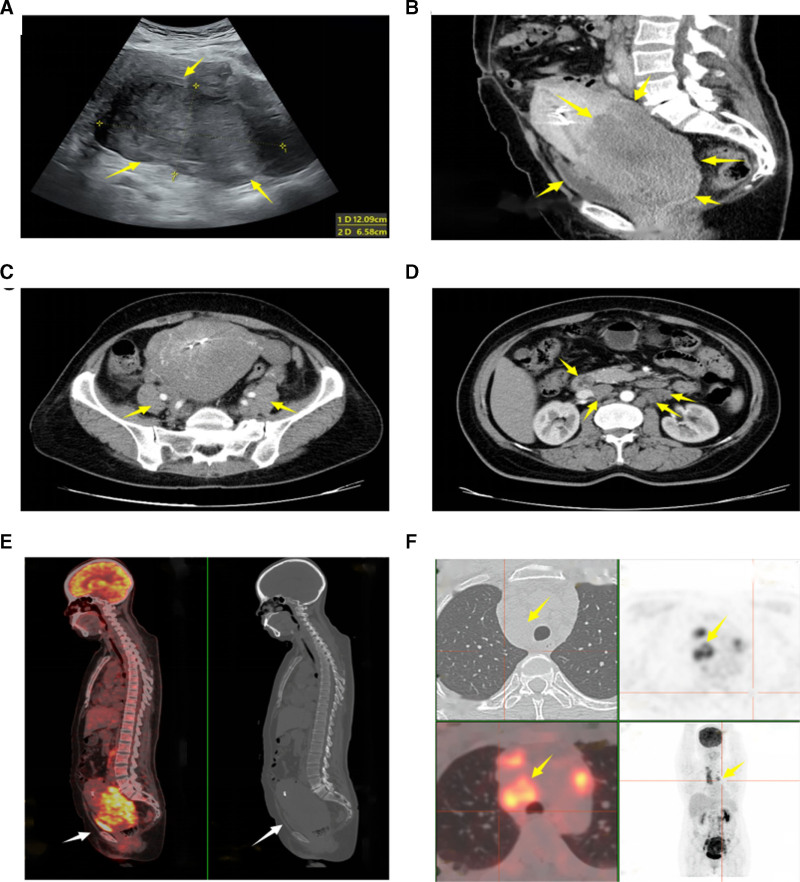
Imaging indicated the mass and lymph nodes. (A) Ultrasound showed a hypoechoic lesion extending from the anterior uterine wall to the cervix. (B–D) Computed tomography scan showed enlarged: cervix, uterus (B), pelvic lymph nodes (C) and para-aortic lymph nodes (D). (E and F) PET/CT demonstrated the mass in the pelvic cavity (E) and metastasis of mediastinal lymph nodes (F). CT = computed tomography, PET = positron emission tomography.
